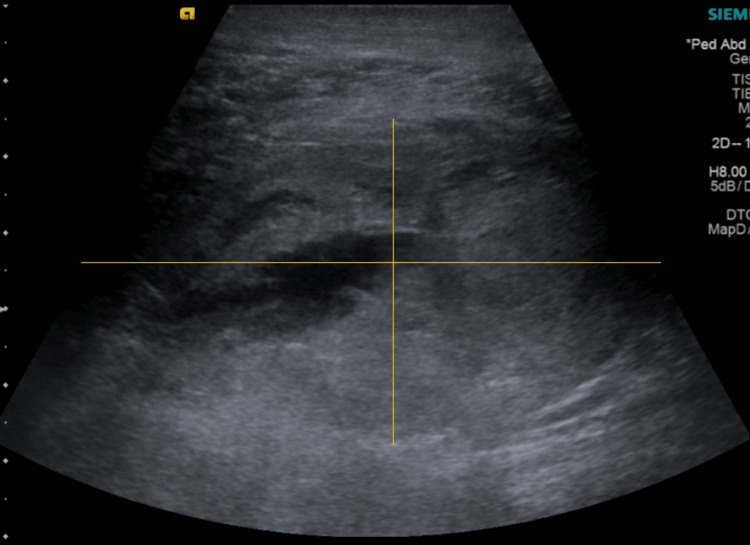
Ultrasound imaging displaying a large hematoma in the superficial posterior compartment.
